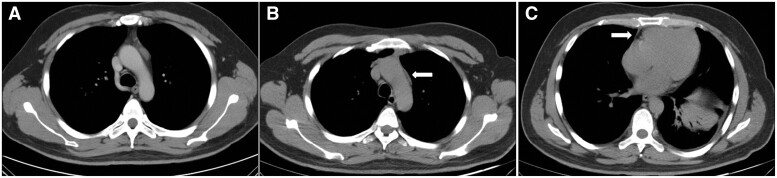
Computed tomography showed residual fat after subxiphoid thymectomy. (A) Thymoma was indicated before surgery; (B) the fat adjacent to the aortic arch; and (C) the soft tissue located in the cardiophrenic angle.
